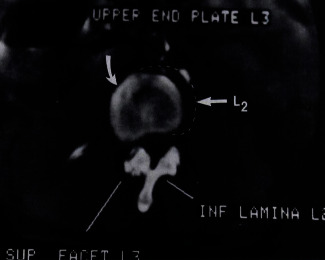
An axial scan of a spinal injury by computed tomography (CT) at the 2^nd^ and 3^rd^ lumbar vertebrae.
